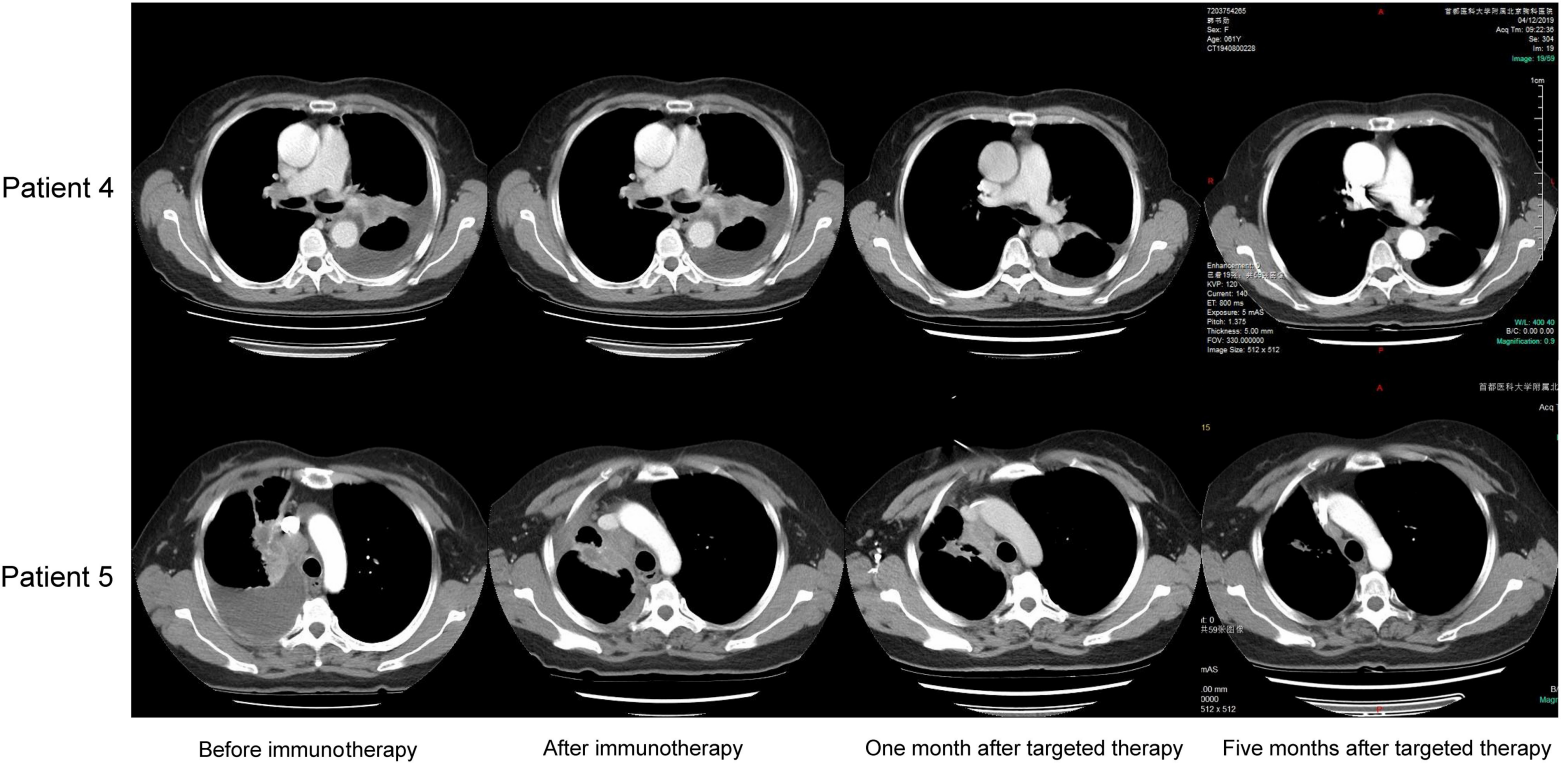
Representative images from chest CT.
